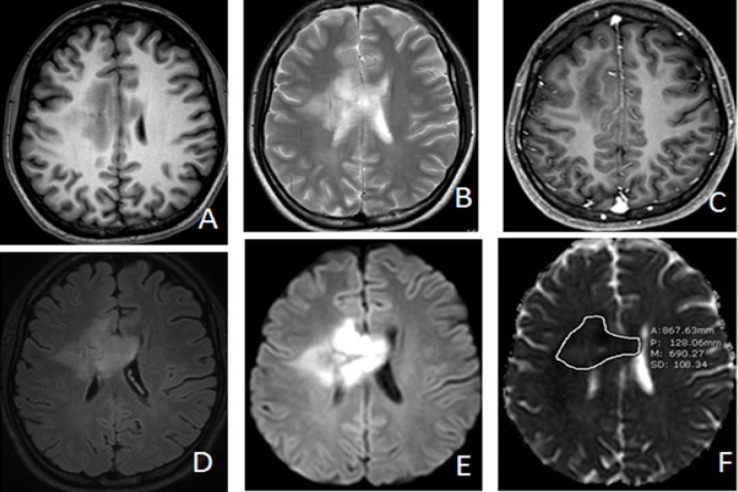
Anaplastic Astrocytoma (WHO Grade III) in 19 Year-Old-Woman. Axial T1W (A), T2W(B), Post contrast T1WFS(C), FLAIR (D), DWI(E) and ADC map (F) images representing case that showed marked hyperintense solid part of the tumor with visual scale 5 on DWI (E) and ADC value measurement = 690.27 x 10^-6^ mm^2^/s on ADC map(F) which is below the cutoff value
